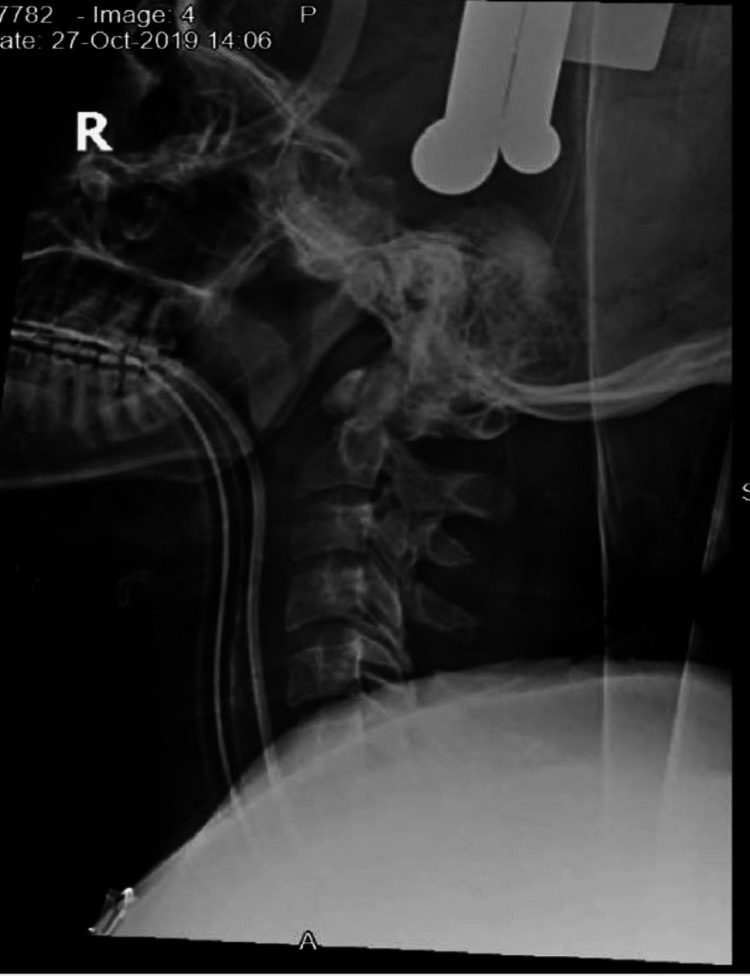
Intra-op lateral cervical X-ray Lateral cervical spine X-ray showing Gardner–Wells traction.
